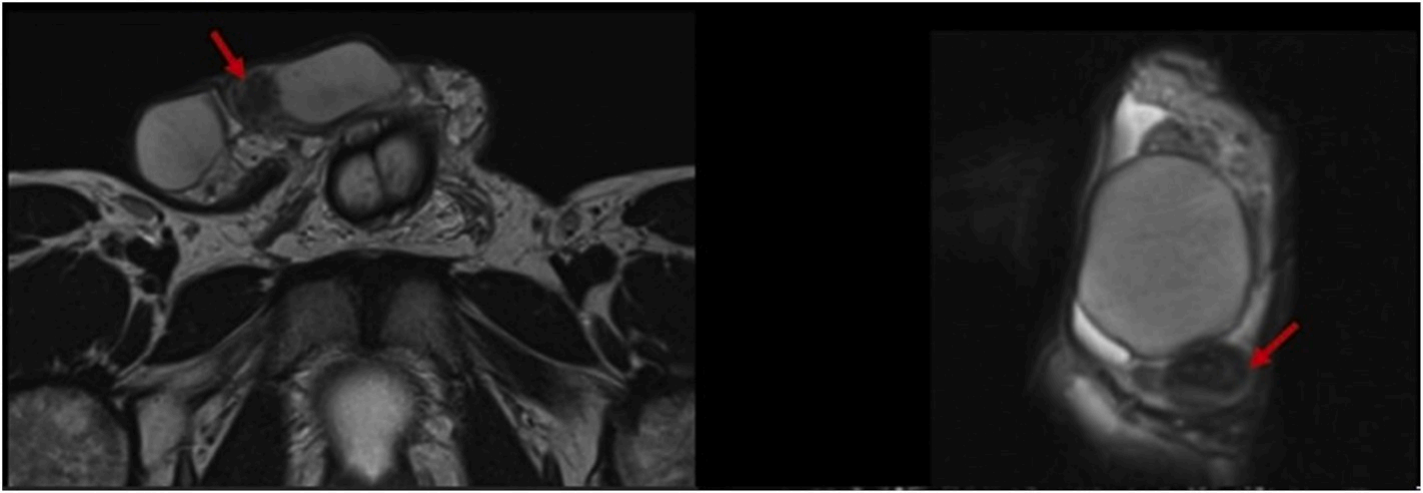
Scrotal MRI: the extra testicular mass appears in relation to the left epididymis tail and display very low T2 signal relative to testicular parenchyma.
